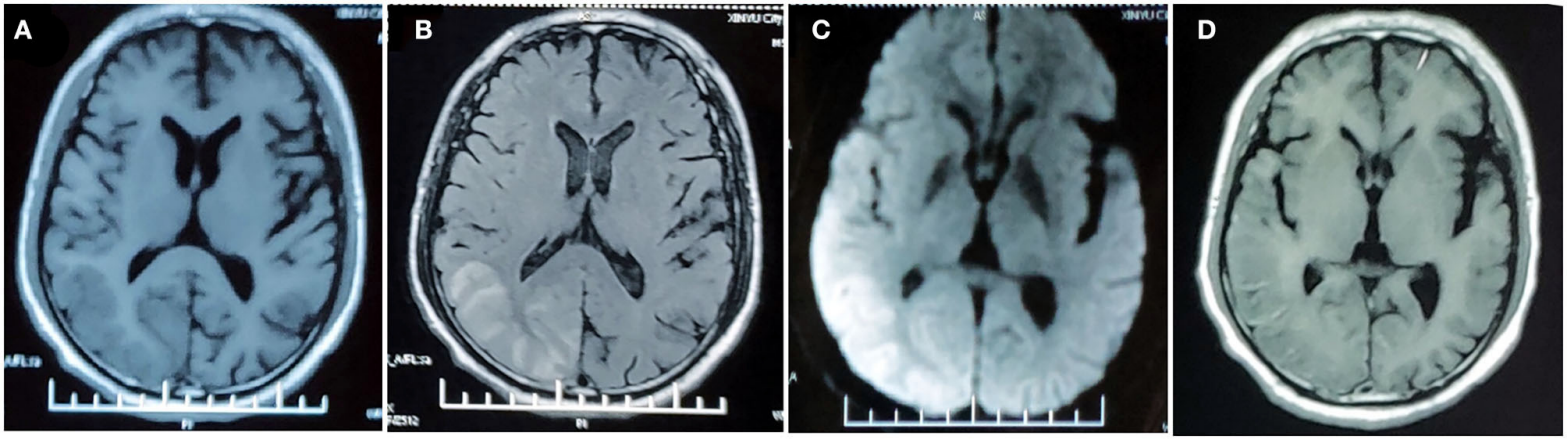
MRI findings in the first prolonged attack. MRI performed 5 days after attack onset demonstrates right temporo-occipital lobe hypointensity on T1-weighted image (A) and hyperintensity on T2-FLAIR image (B) with restricted diffusion on DWI (C). MRI performed 4 weeks after symptom onset demonstrated right temporal–occipital cortical hyperintensity on T1-weighted images (D).
